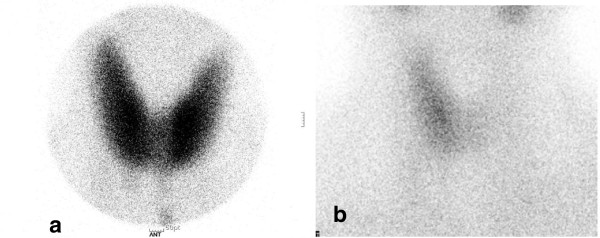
(a) Preoperative Tc-99m scintigraphy checked at a local medical clinic shows normal uptake of the tracer. (b) Postoperative Tc-99m scintigraphy shows faint visualization of the right thyroid lobe.
